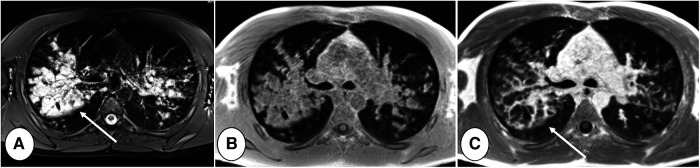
16 years old boy with cystic fibrosis during respiratory tract exacerbation. T2-weighted PROPELLER image (A), T1-weighted (B) pre and post-contrast (C) gradient-echo acquisitions, performed on 1.5 T MRI system (MAGNETOM Avanto, Siemens Healthineers, Enlargen, Germany). Note that in T2-weighted scan bronchial wall cannot be identified in the large bronchiectasis with mucus plugging in the right upper lobe (A, arrow). Gadolinium injection allows identification of bronchial wall thickening and to distinguish between bronchial wall and mucus plugs (C, arrow).
